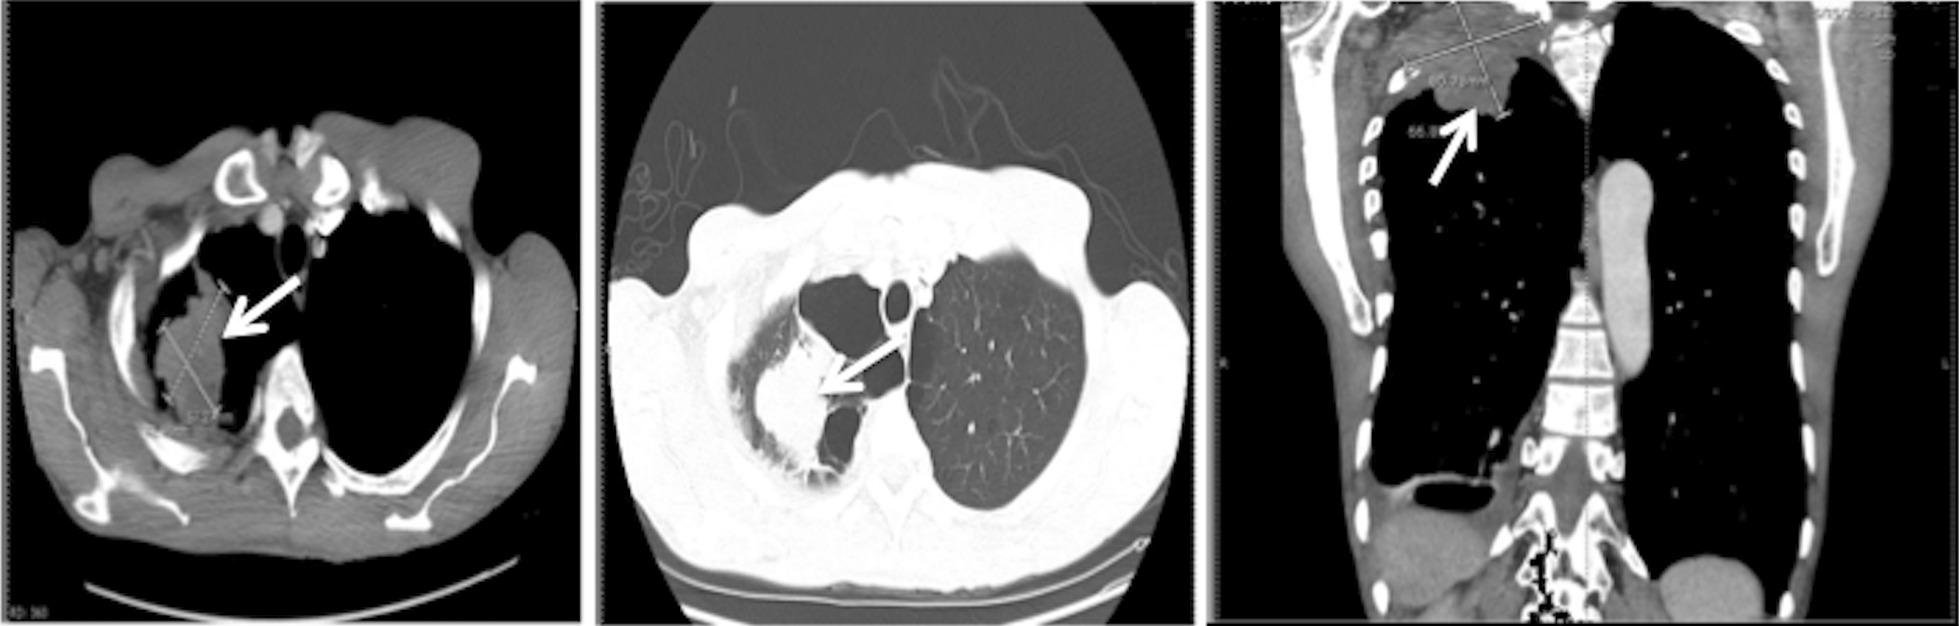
CT images of the chest with and without contrast demonstrating heterogeneous right apical mass causing adjacent rib destruction, extending into the right supraclavicular region (5.2 × 7.6 cm^2^), most likely Pancoast tumor (arrowhead and crosses on CT images)
